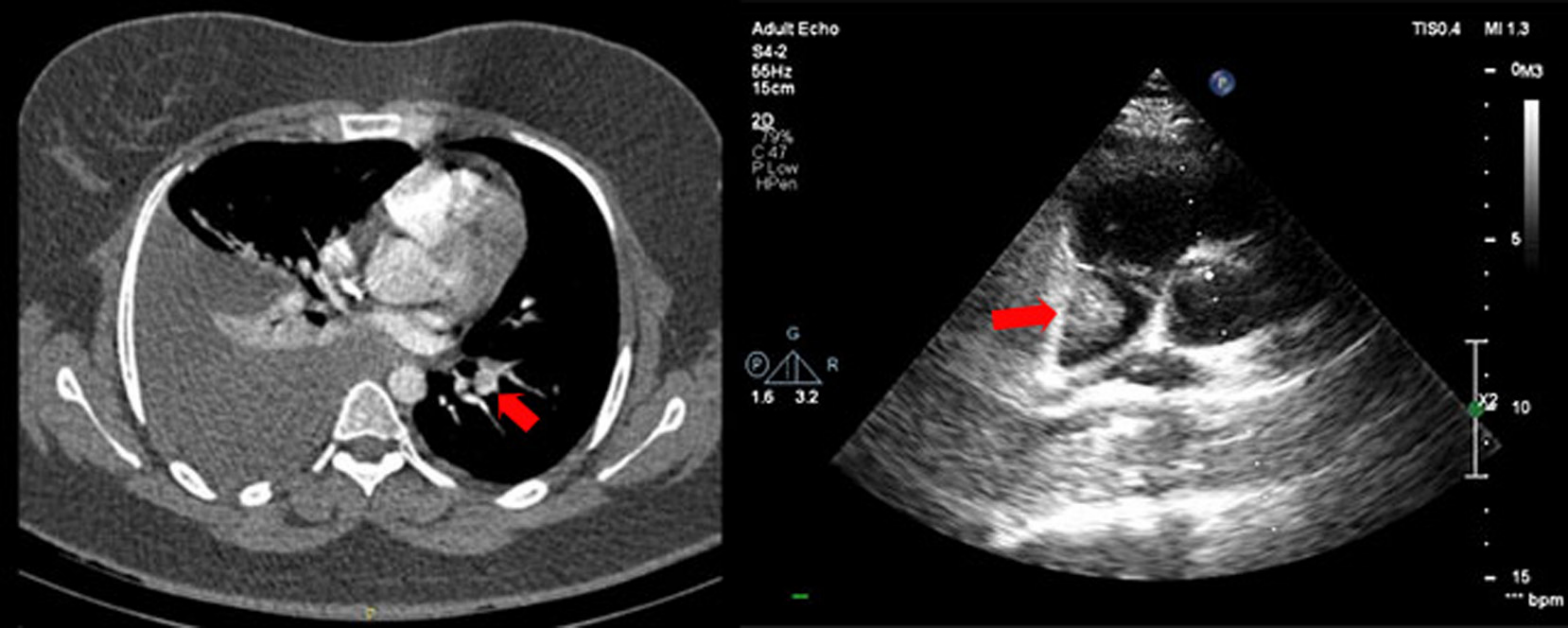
CTPA (left): The red arrow shows the clot in one of the branches of the left pulmonary artery. Echocardiography (right): The red arrow shows the large thrombus in the right atrium.
